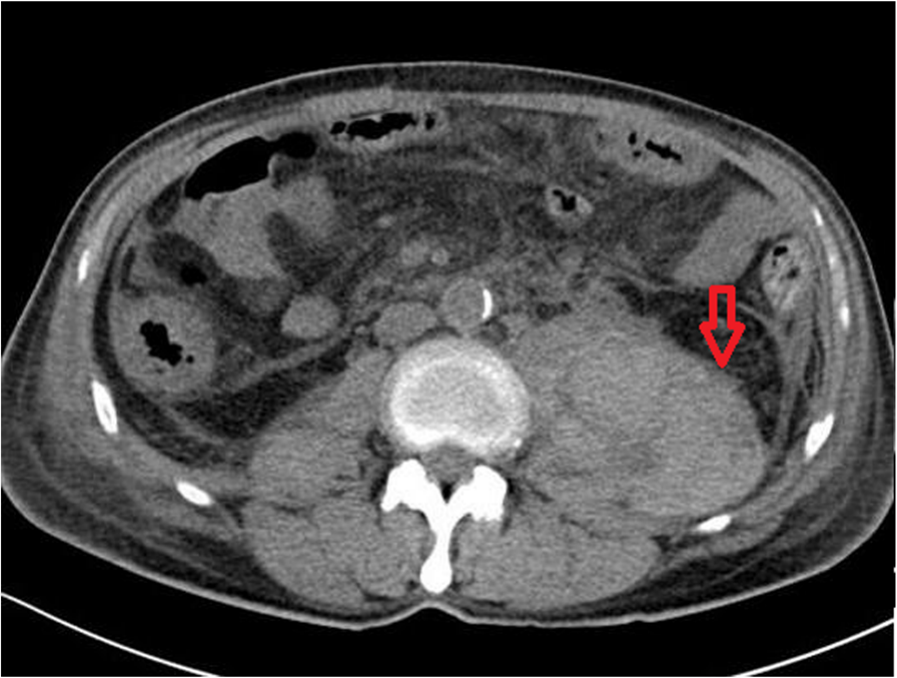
Radiographic imaging revealed a massive hematoma of the left psoas major muscle (arrow) (Enhanced abdominal CT was showed in Additional file 3)
